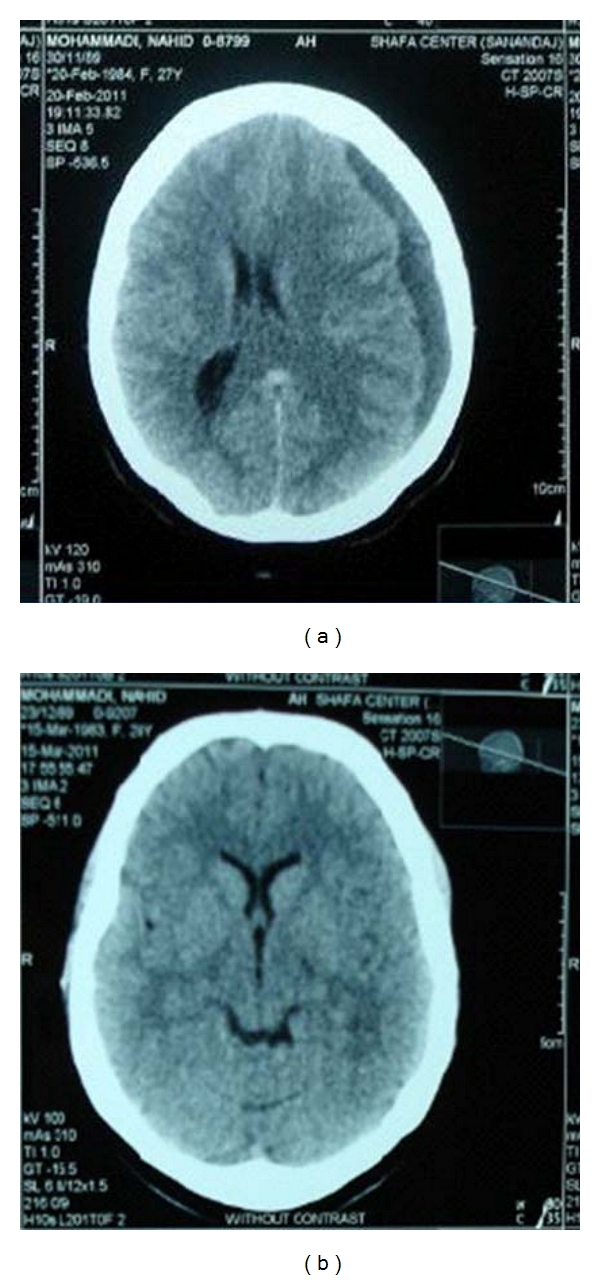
(a) Brain computed tomography scan showed a late subacute SDH of the left frontotemporal region and midline shift. (b) Normal control Computed tomography scan.
